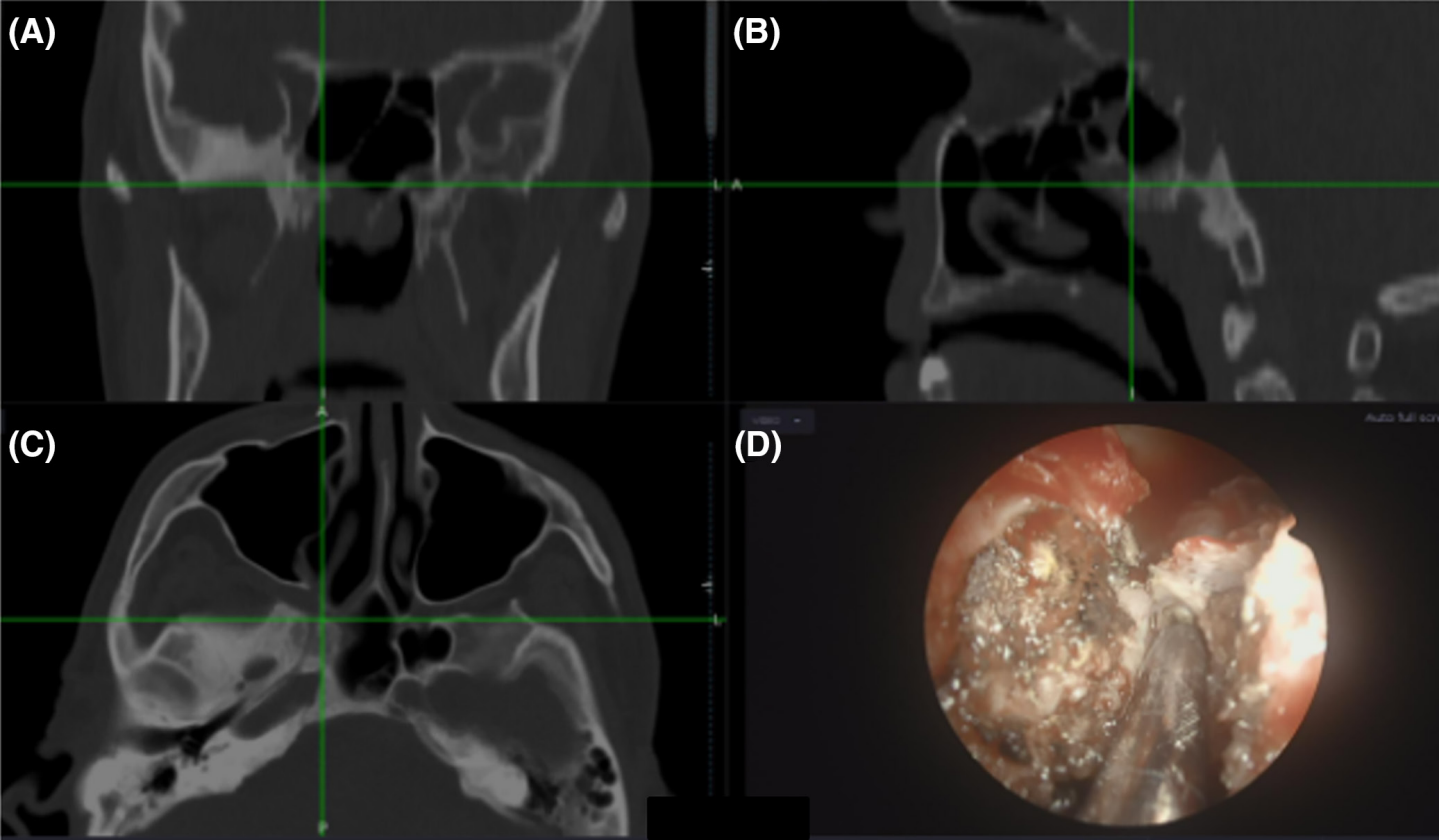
Image‐guided navigation system used reformatted thin‐cut CT scans in coronal (A), sagittal (B), and axial (C) sections to create a three‐dimensional portal. The neoplasm invaded the posterior wall of the right pterygopalatine fossa (A–C). A friable tumor is separated from the structures of the right pterygopalatine fossa as shown in the endoscopic image (D).
